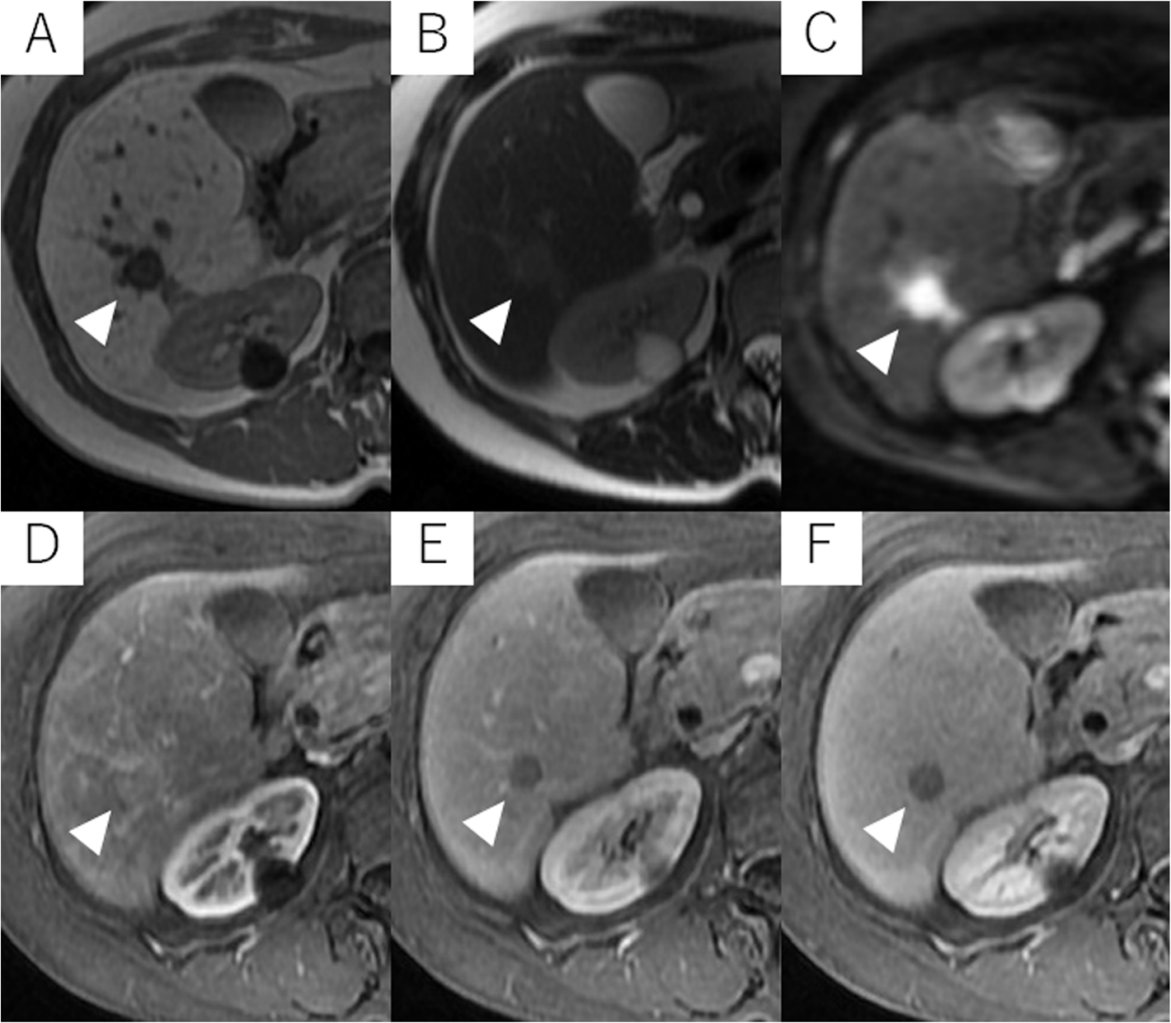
MRI demonstrates that the nodule shows well-defined low signal intensity in T1-weighted image (a), slightly high signal intensity in T2-weighted image (b), and strongly high signal intensity in diffusion-weighted image (c). Gd-EOB-DTPA-enhanced MRI reveals that the nodule is slightly enhanced in the arterial phase (d). The lesion shows perinodular enhancement in the portal phase (e) and a defect in hepatobiliary phase (f) (white arrowhead)
